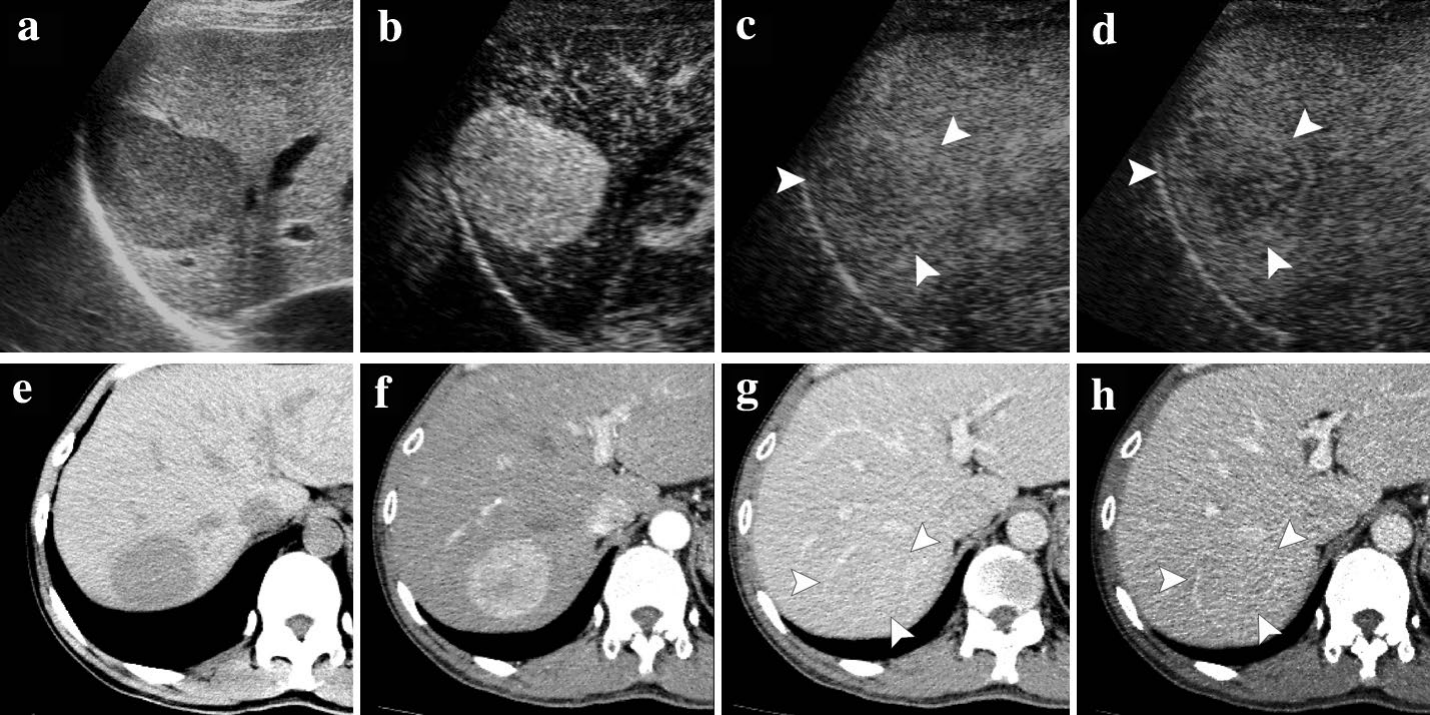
Contrast-enhanced ultrasound (CEUS) and dynamic computed tomography (CT) images of HCA in a 41-year-old female (Case 3) demonstrate a hypoechoic (a) and hypointense (e) lesion to the surrounding liver. On contrast-enhanced imaging, both modalities showed hyper-enhancement in the arterial phase (b, f). During the portal and late phases on CEUS, the lesion showed hypo-enhancement (c, d). However, the lesion is iso-enhanced (g, h)
